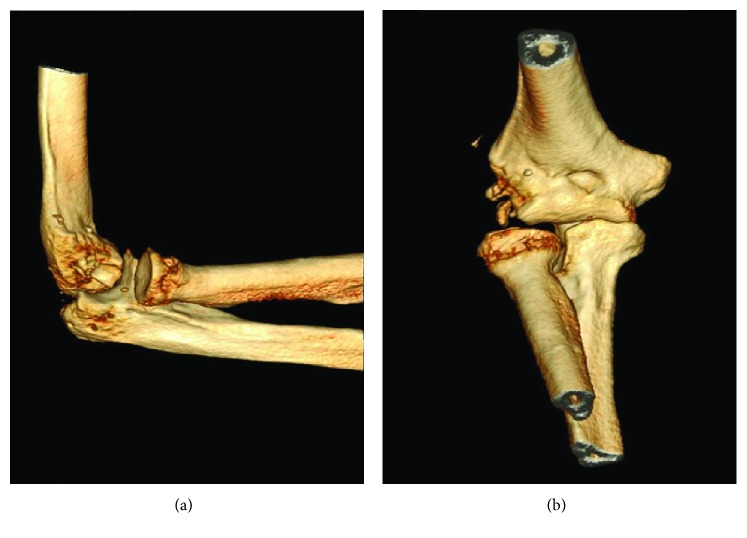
(a) Lateral view and (b) anterior view of preoperative *3-D CT scan* reconstruction showing external condyle loss and radial head subluxation.
